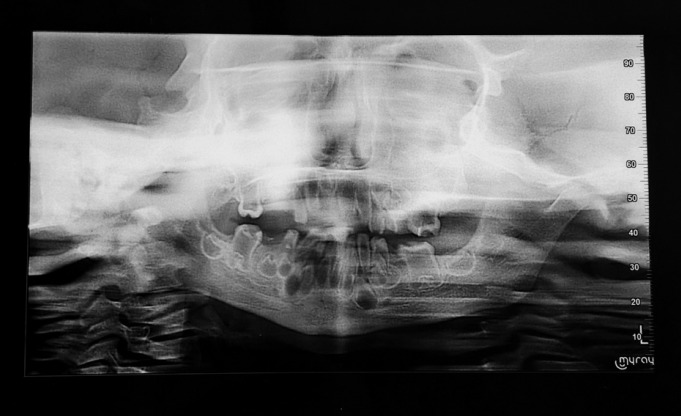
Panoramic radiograph showing taurodontic teeth and thickened cortical bone.
